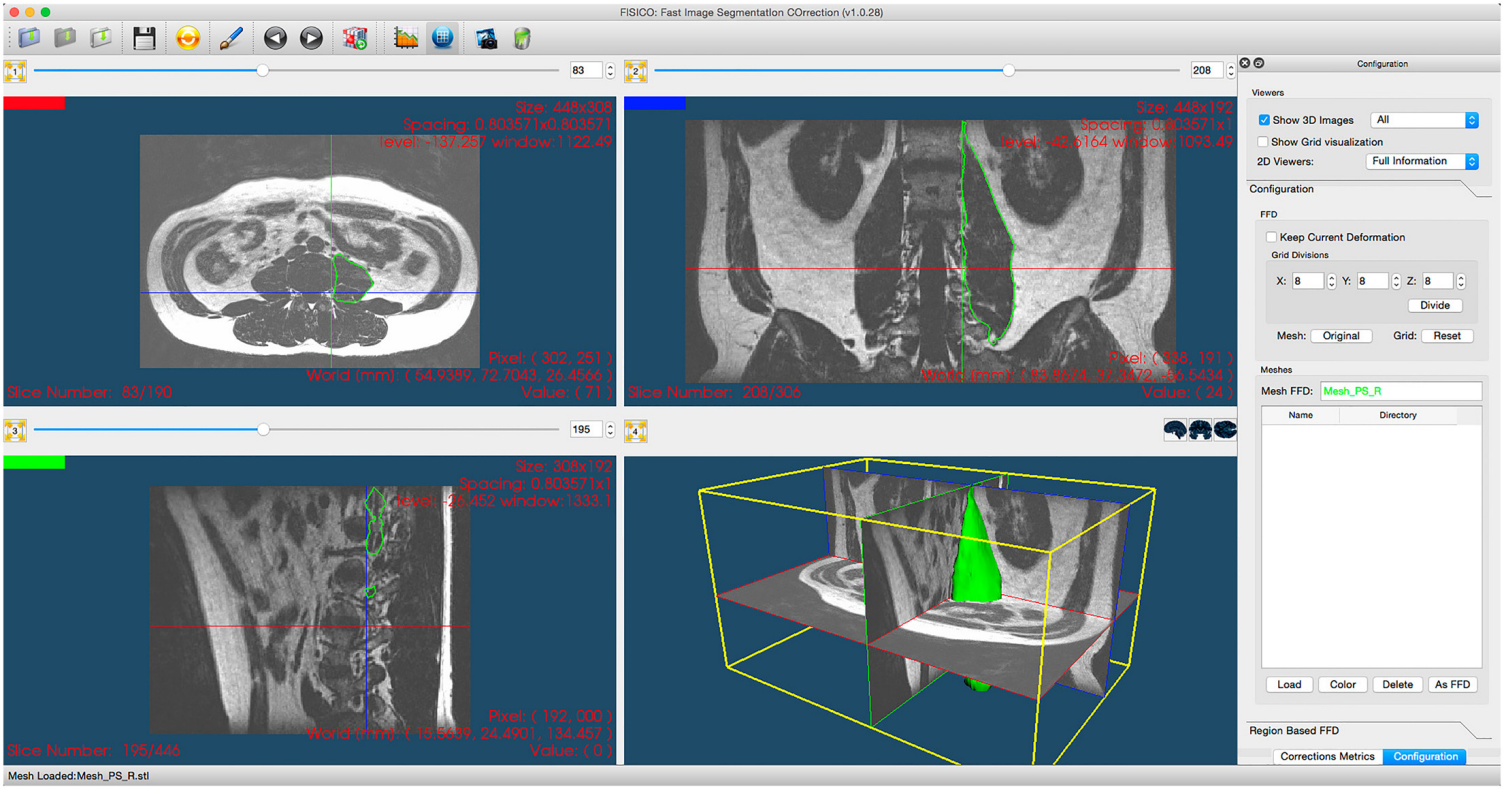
Correction software. The center part shows three 2D viewers with MR image planes and contours of the input segmentation, the third panel shows 3D environment. The right side is optional and shows the corrections metrics: Correction Time, Dice coefficient and user information. Also, contains the controls of the local and full correction modes.
